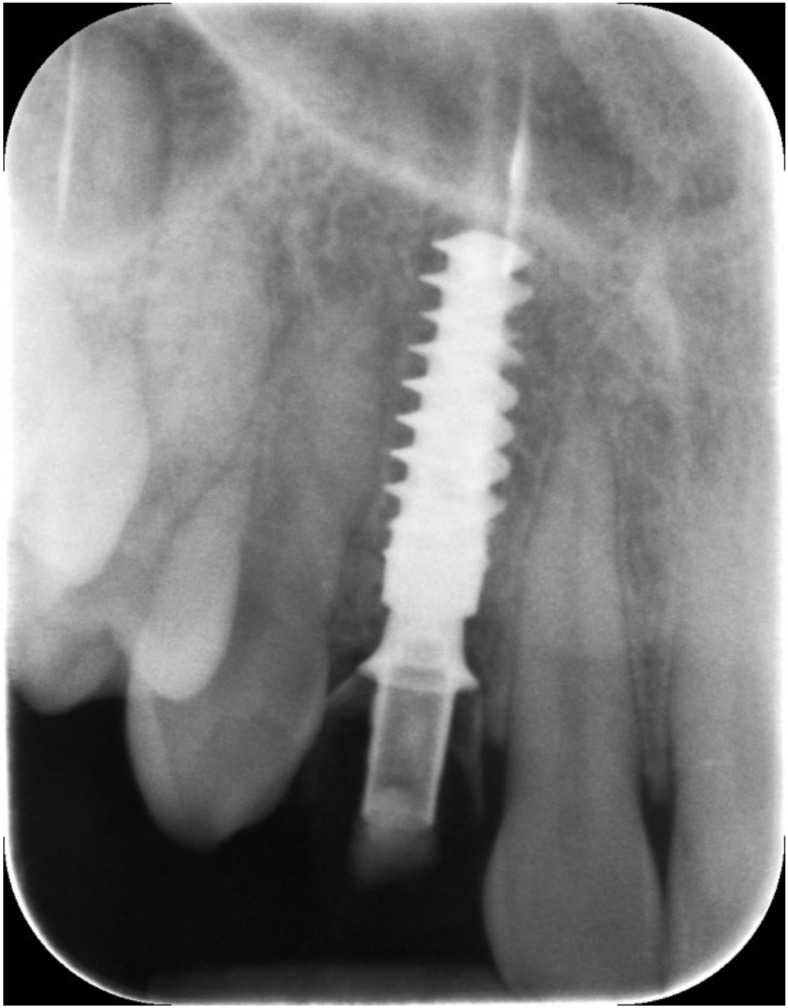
Periapical X-ray at immediate temporization.
